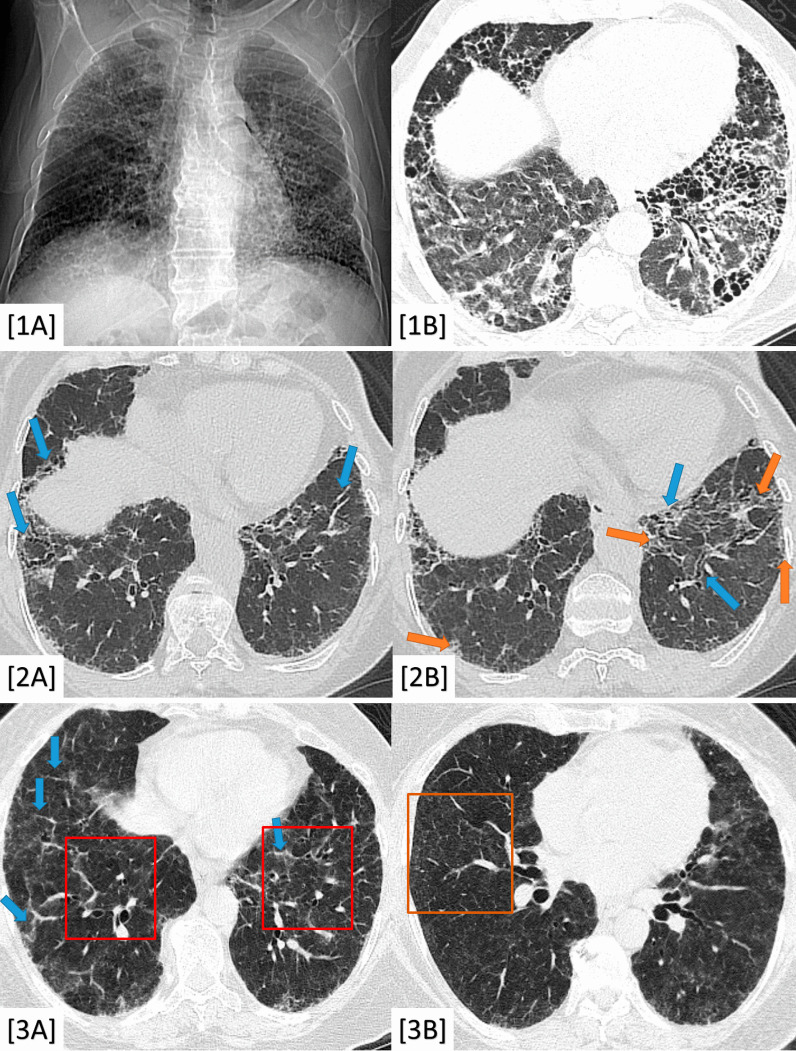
1A, B A 55-year-old male patient complaining of progressive dyspnea with restrictive pulmonary functions; 1A X-ray chest PA view, 1B Axial chest CT (lung window); both showing extensive fibrotic changes and honeycombing bronchiectasis, more pronounced in lower lung zones. Diagnosis: Proved patient with UIP/IPF. 2A, B A 53-year-old female patient with a history of rheumatoid arthritis who was complaining of progressive dyspnea (2A, B) Axial chest CT (lung window); both showing bilateral lower lobar mosaic ground-glass attenuation (orange colored arrows) mixed with peri-bronchial thickening and traction bronchiectasis/bronchiolectasis (blue colored arrows) without honeycombing. Diagnosis: Proved patient with fibrosing NSIP. 3A, B A 43-year-old female patient complaining of progressive dyspnea with a positive history of raising birds. 3A Axial chest CT (lung window) showing “head-cheese sign” (red colored squares) and fine septal thickening (blue colored arrow). 3B Axial chest CT (lung window) showing faint centrilobular ground glass nodules (example: orange colored square). Diagnosis: Proved patient with mixed sub-acute and chronic hypersensitivity pneumonitis (HP)
